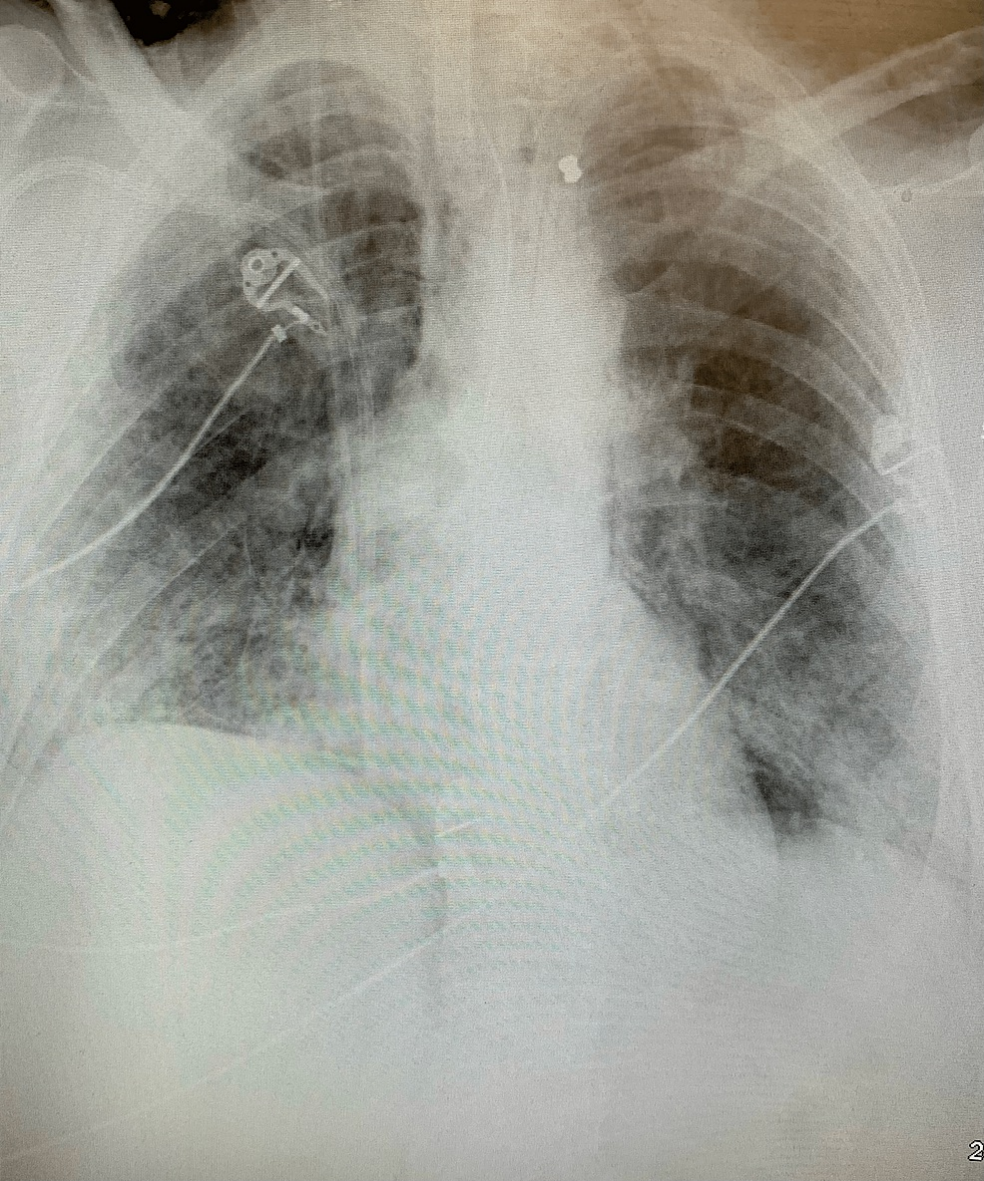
Chest X-ray showing no pneumothorax in the patient.
